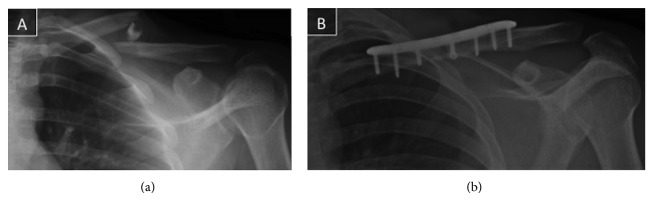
21-year-old male patient with basketball trauma. (a) He presents a type-2B-1 fracture. (b) Immediate postoperative radiographs. Reduction and osteosynthesis with an 8-hole precontoured locking plate using 6 screws, 3 lateral and 3 medial to focus.
